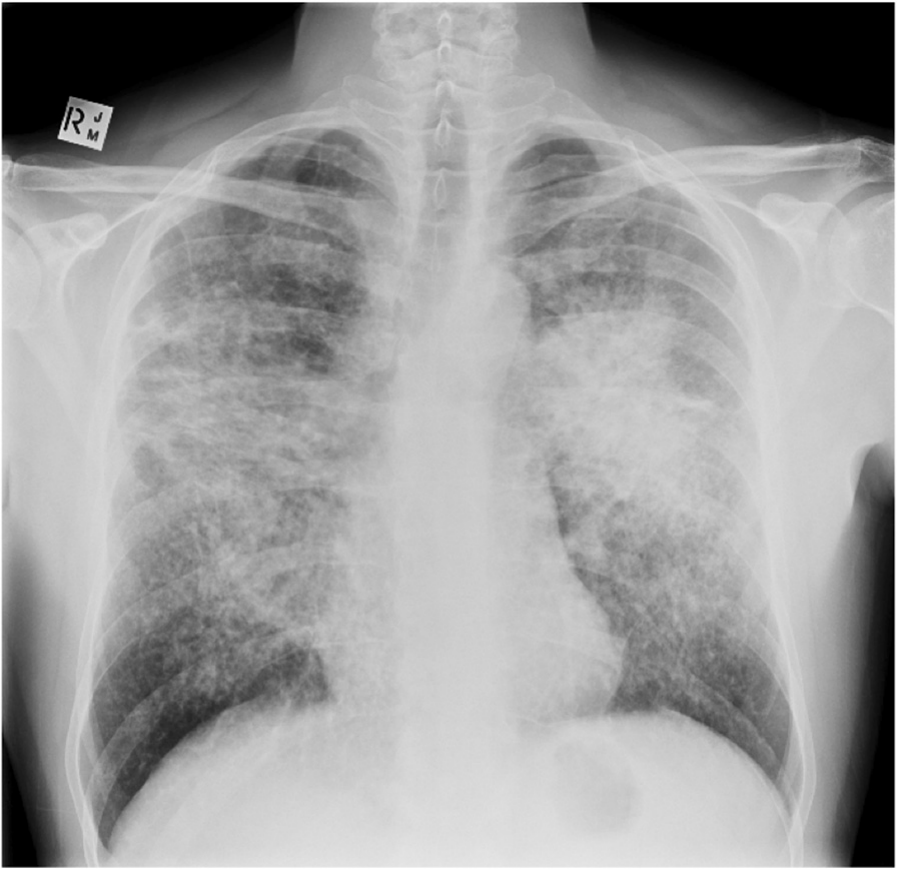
A frontal chest x-ray showing changes consistent sarcoidosis. There is bilateral bulky hilar and paratracheal lymphadenopathy together with extensive, confluent reticulonodular opacities with a characteristic distribution in the mid and upper zones.
